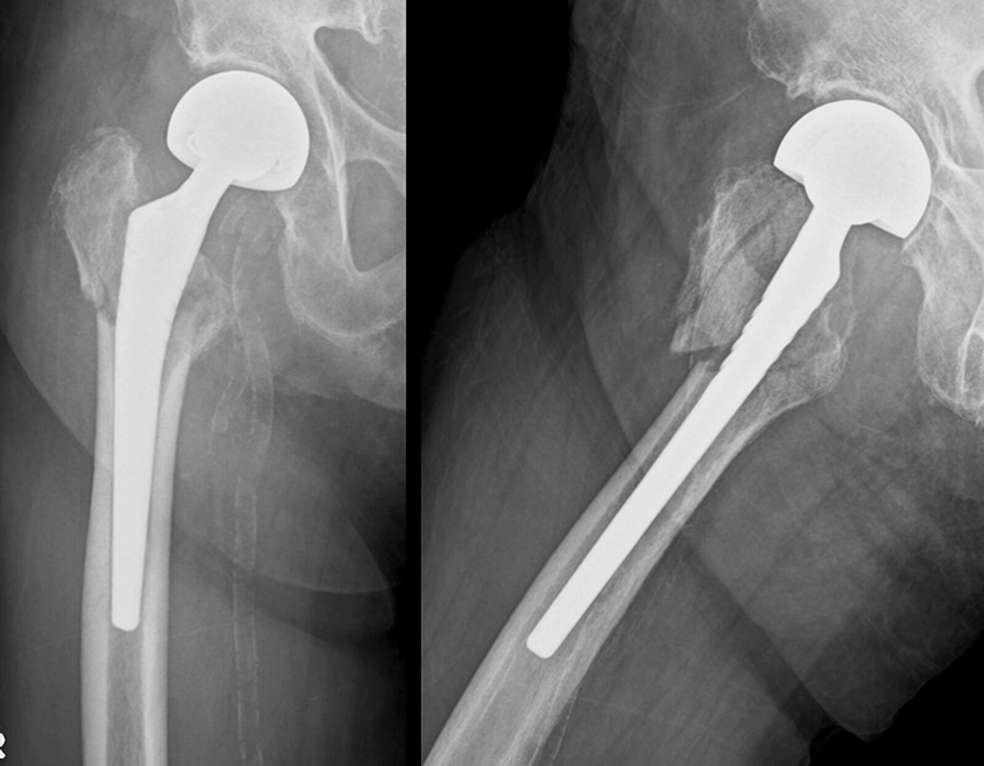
Preoperative radiographs of case 2 Preoperative radiographs of case 2 showed PFF of Vancouver classification Type B1. PFF: periprosthetic femoral fracture.
